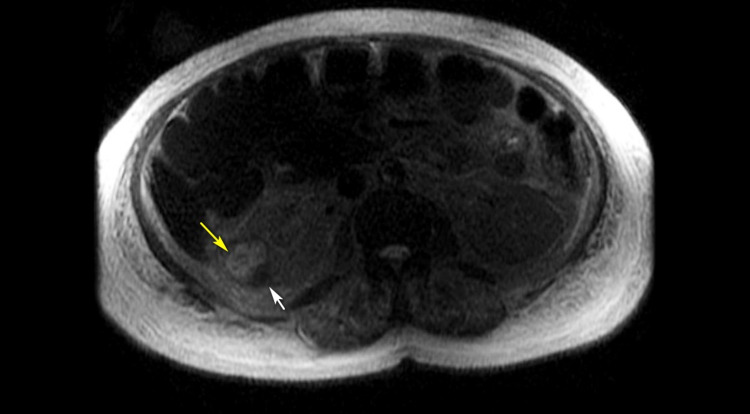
MRI of the abdomen and pelvis. A 3-cm heterogeneous signal lesion exophytic from the lateral portion of the mid-right kidney was observed (yellow arrow). A cystic lesion was found adjacent to this lesion and slightly inferior, suggestive of a hemorrhagic cyst (white arrow). MRI: magnetic resonance imaging
